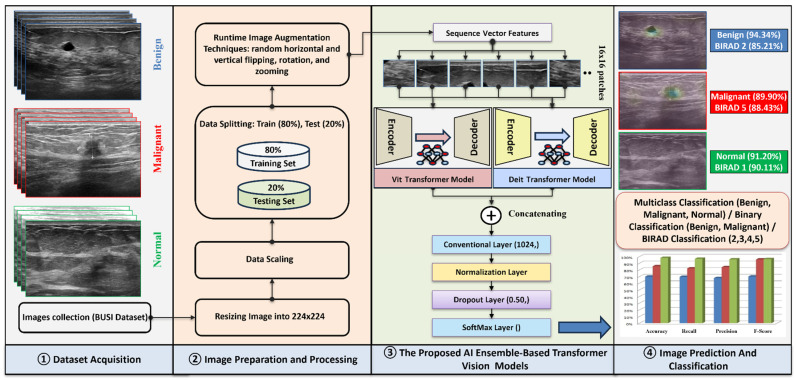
The proposed ensemble-based Vision Transformer model for breast cancer classification using ultrasound images.
